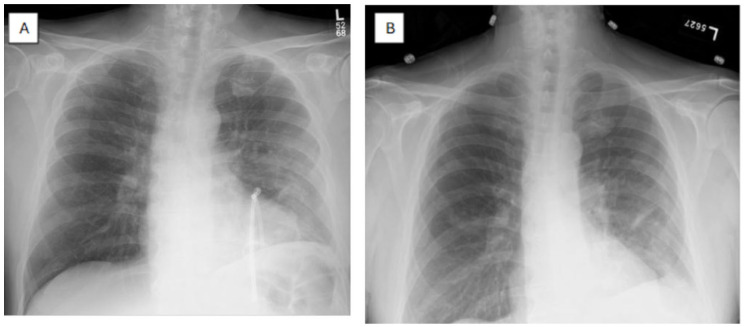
(a) CXR showing left basilar pleural drain appears to be kinked. Small residual left pleural effusion. There is upward displacement of the left hemidiaphragm, likely due to the adjacent pulmonary abcess. (b) Follow up CXR shows minimal pleural fluid remaining after removal of the left chest tube with subsegmental atelectasis in the left lung base. The right and upper left lungs are clear, and the heart and mediastinum are unremarkable. CXR: Chest radiography.
